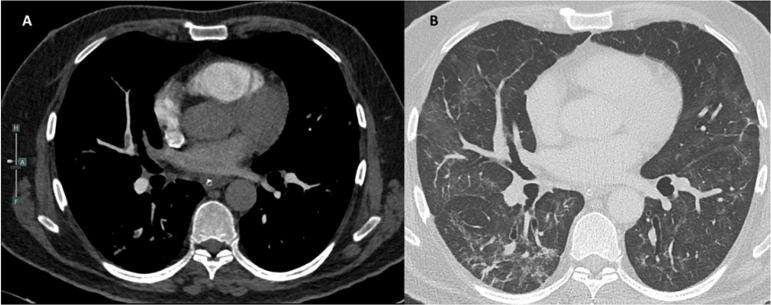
Pulmonary computed tomography of a patient from the studied sample. A filling defect of the segmental branch of the middle lobe pulmonary
